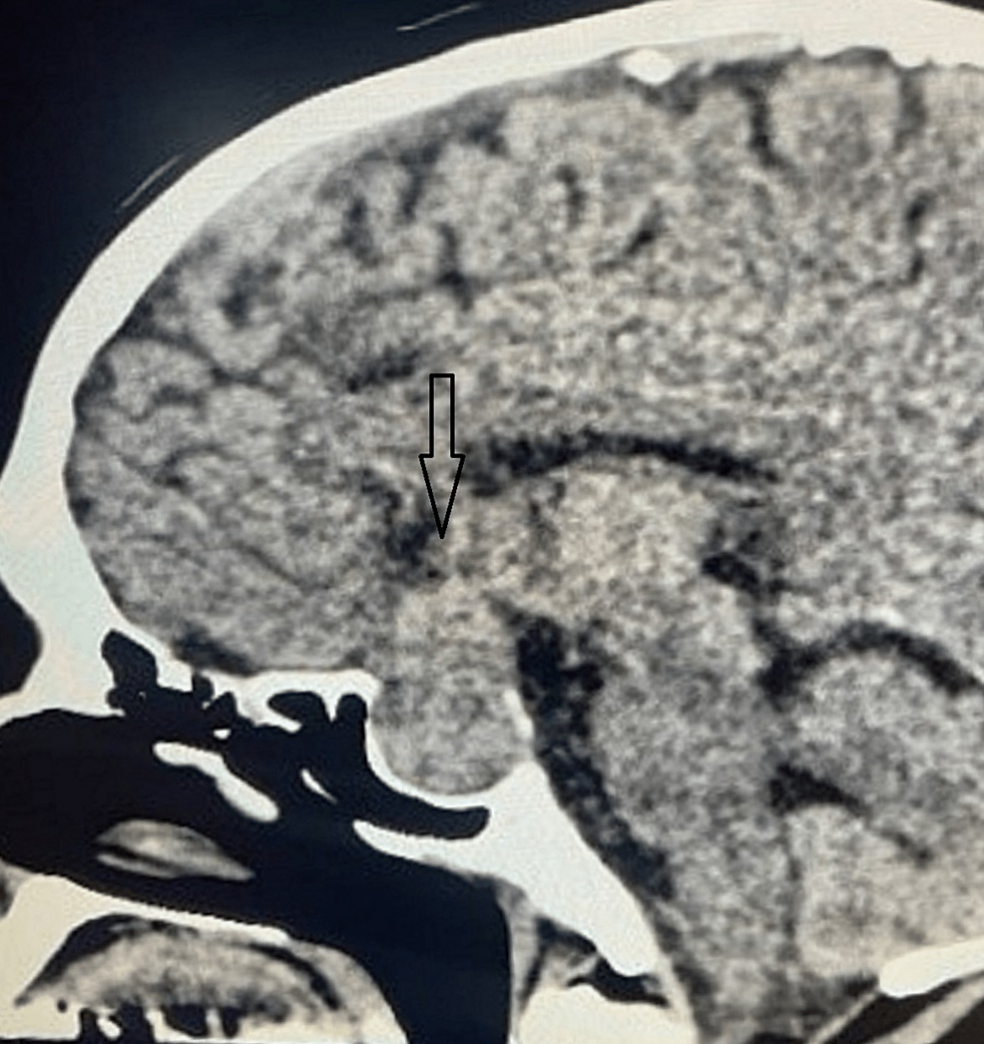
CT scan sagittal view showing sellar and suprasellar mass lesions.
